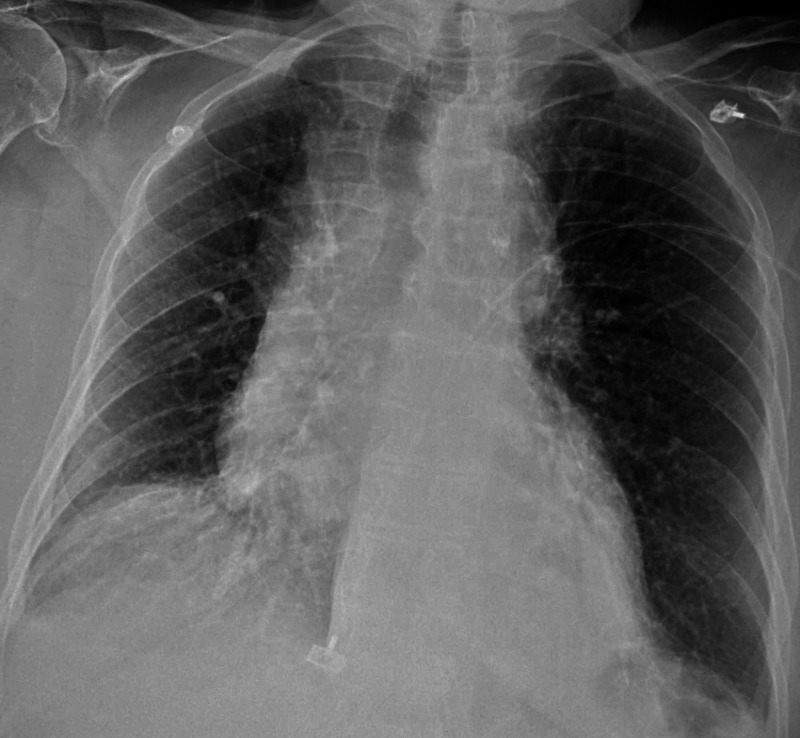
Chest X-ray did not reveal any infiltrate or effusion.
